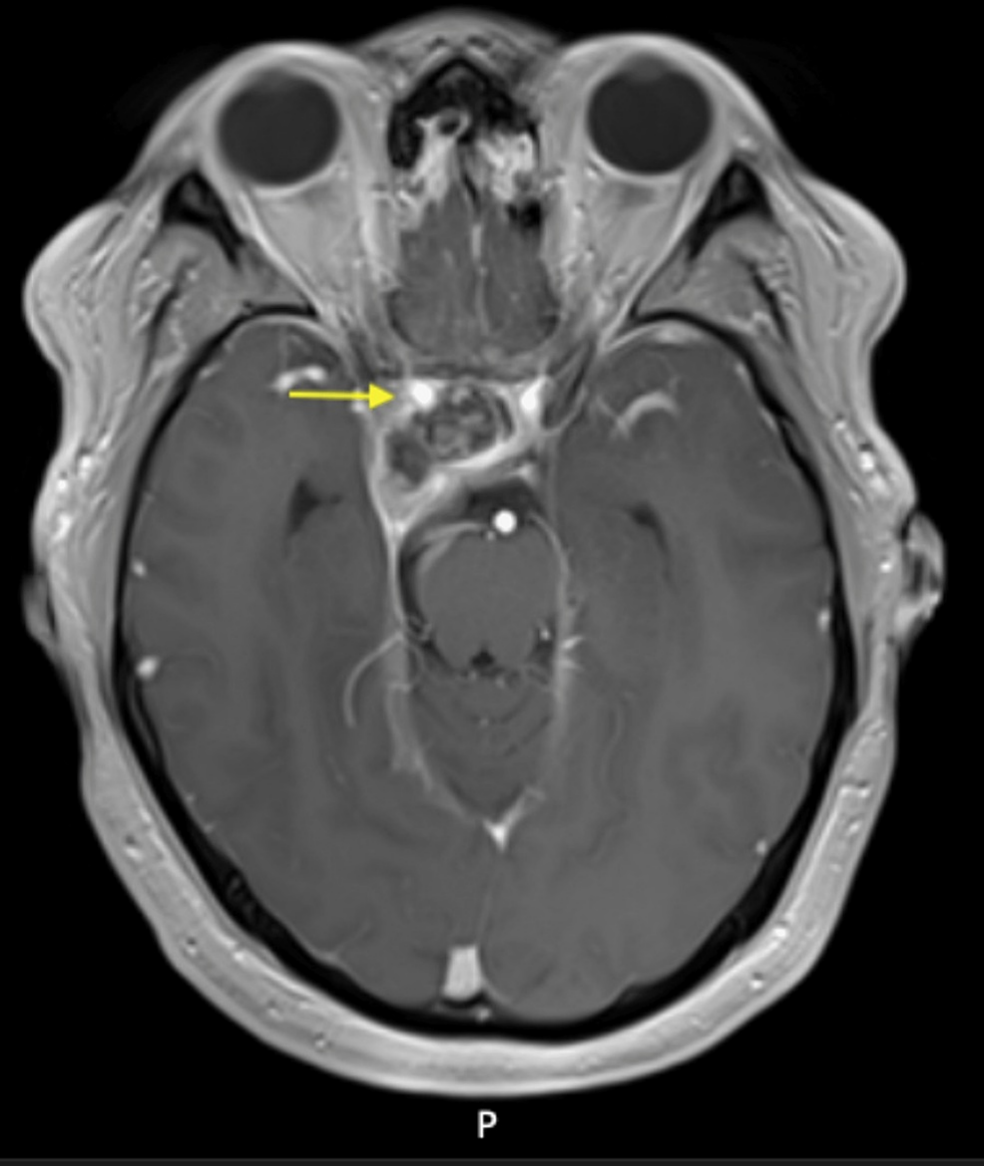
MRI brain axial T1 post-contrast showing right internal carotid artery (yellow arrow) with residual tumor and post-surgical changes.
